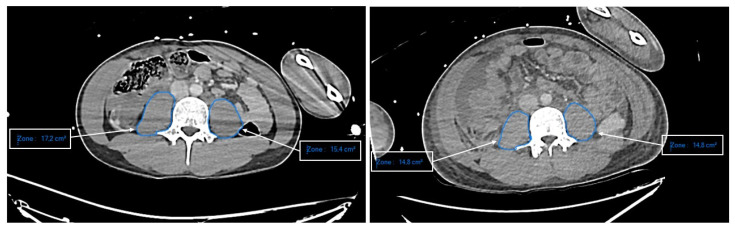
Example of muscle psoas cross-sectional area (CSA) measurement on the first (day 1) and the second (day 4) abdominal CT scan. Zone: Muscle psoas cross-sectional area calculated at the L4 level. Mean CSA at day 0: 1630 mm^2^; mean CSA at day 4: 1480 mm^2^. ΔCSA = 375 mm^2^/day; %ΔCSA = 5.8% per day.
